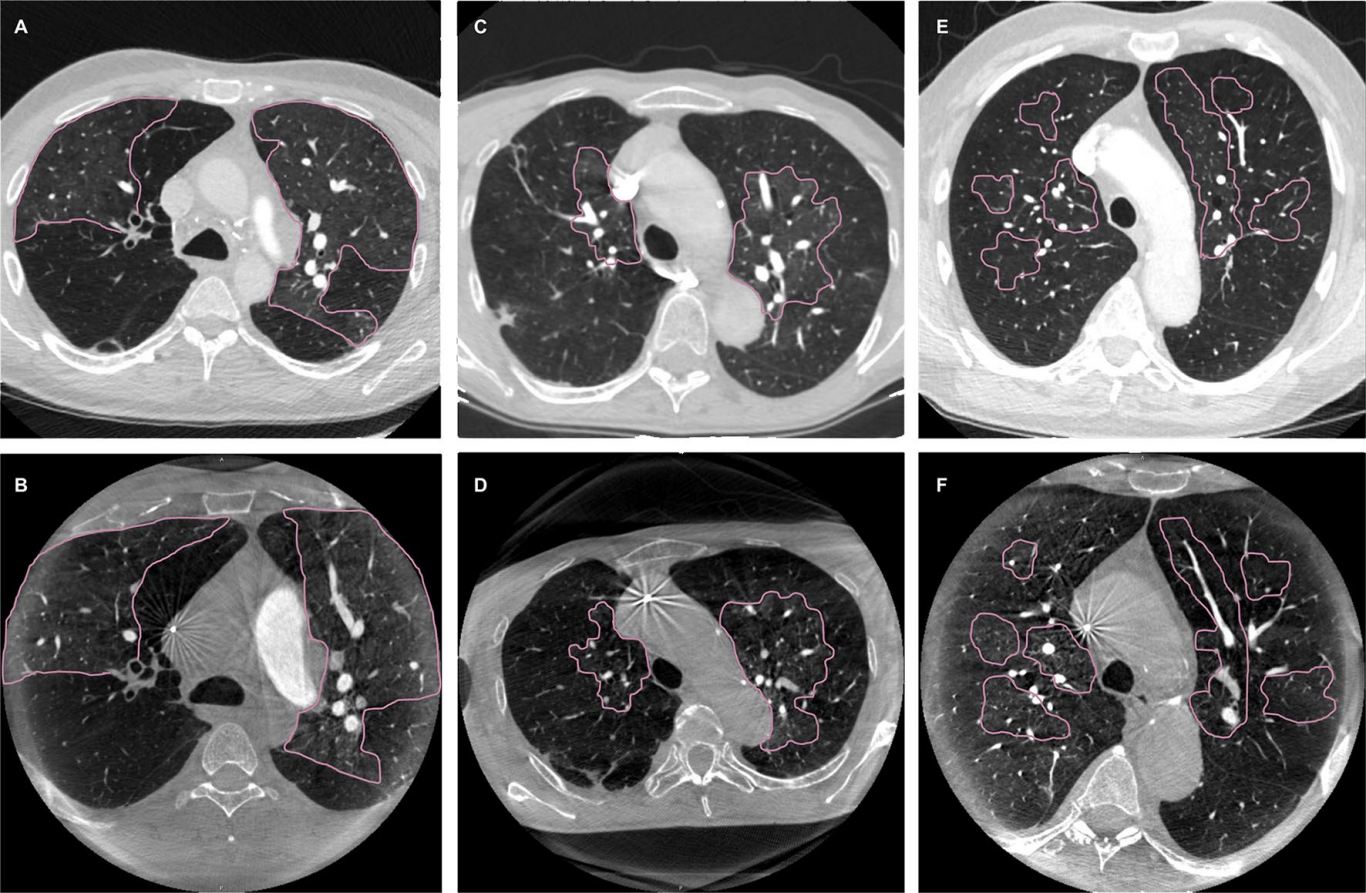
Patterns of mosaic perfusion on CT and CACT. (A, B) pattern 1, sharply demarcated segmental and/or subsegmental areas of hypo- and hyperattenuation with well-defined borders corresponding to the anatomic unit of the secondary pulmonary lobule on CT (A) and CACT (B); (C, D) pattern 2, perihiliar hyperattenuating areas with peripheral perfusion defects on CT (C) and CACT (D); (E, F) pattern 3, diffuse heterogenity of lung attenuation with patchy low-attenuating areas located more centrally within in the secondary lobule and intermixed with areas of normal or increased attenuation on CT (E) and CACT (F).
